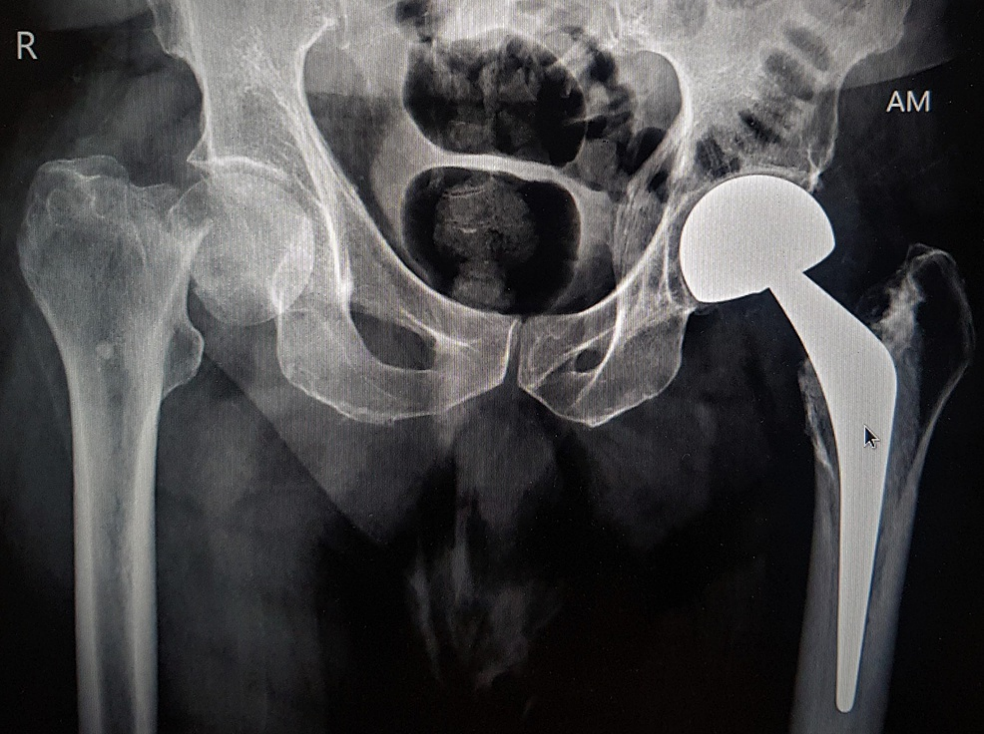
Pelvis radiograph with a right hip fracture of the neck femur and left hip bipolar prosthesis in situ
